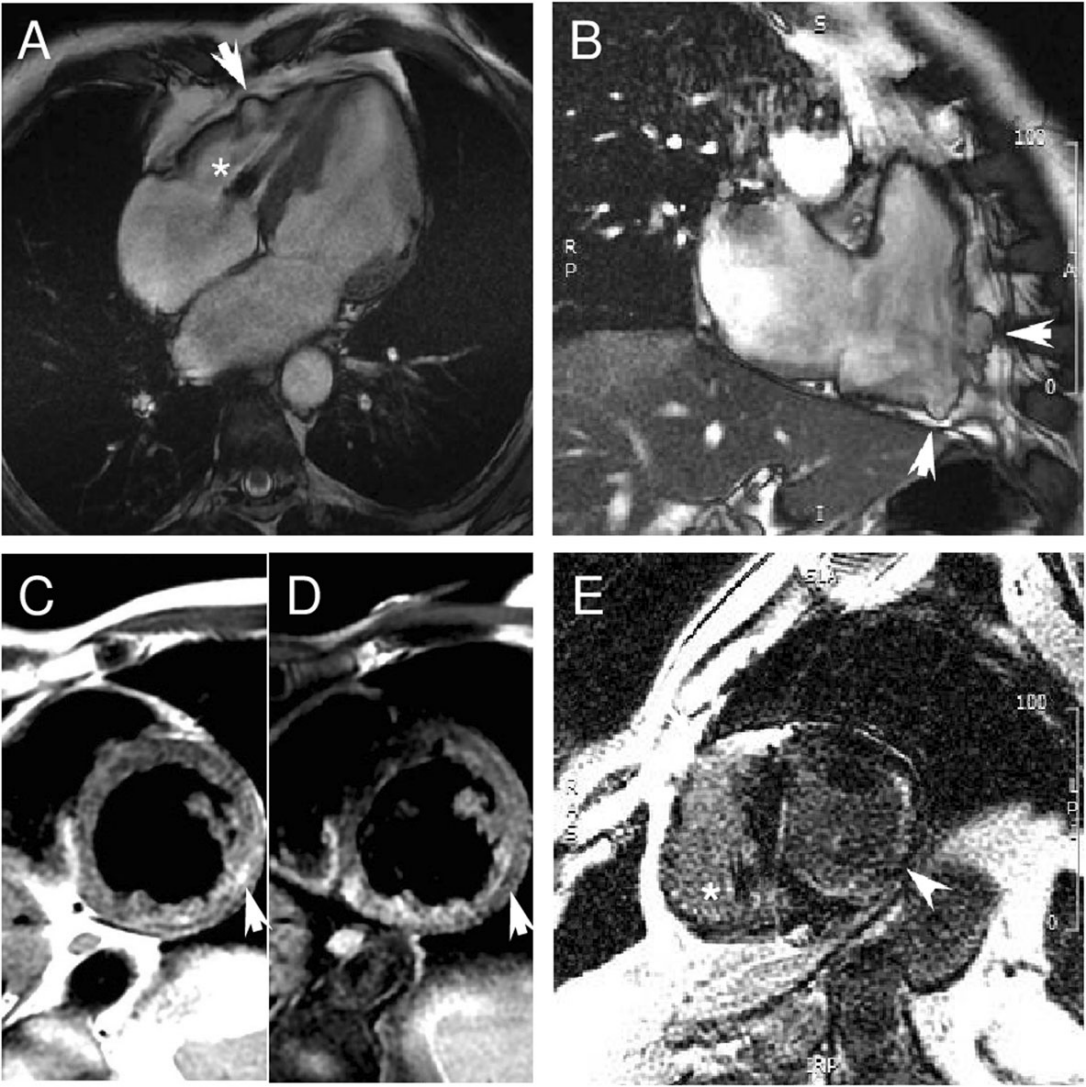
CMR of a 54-year male, carrier of p.Arg401His missense *LMNA* mutation, affected by DCM. (a-b) Four chamber and RV long axis SSFP images show biventricular dilation, bulging of the RV free wall (white arrow, panel a-b) and diaphragmatic wall (white arrow, panel b). (c) Two chamber T1-weighted and (d) fat suppressed T1-weighted slices showing LV fatty replacement of mid lateral wall (white arrow). (e) IR LGE slice showing fibrosis in the infero-lateral wall (with focal transmural pattern) and in the interventricular septum (image quality was due to respiratory artifacts and to the presence of pacemaker [*]). CMR: cardiac magnetic resonance. DCM: dilated cardiomyopathy. SSFP: steady-state free precession. LV-RV: left-right ventricle. IR LGE: inversion recovery late gadolinium enhancement
